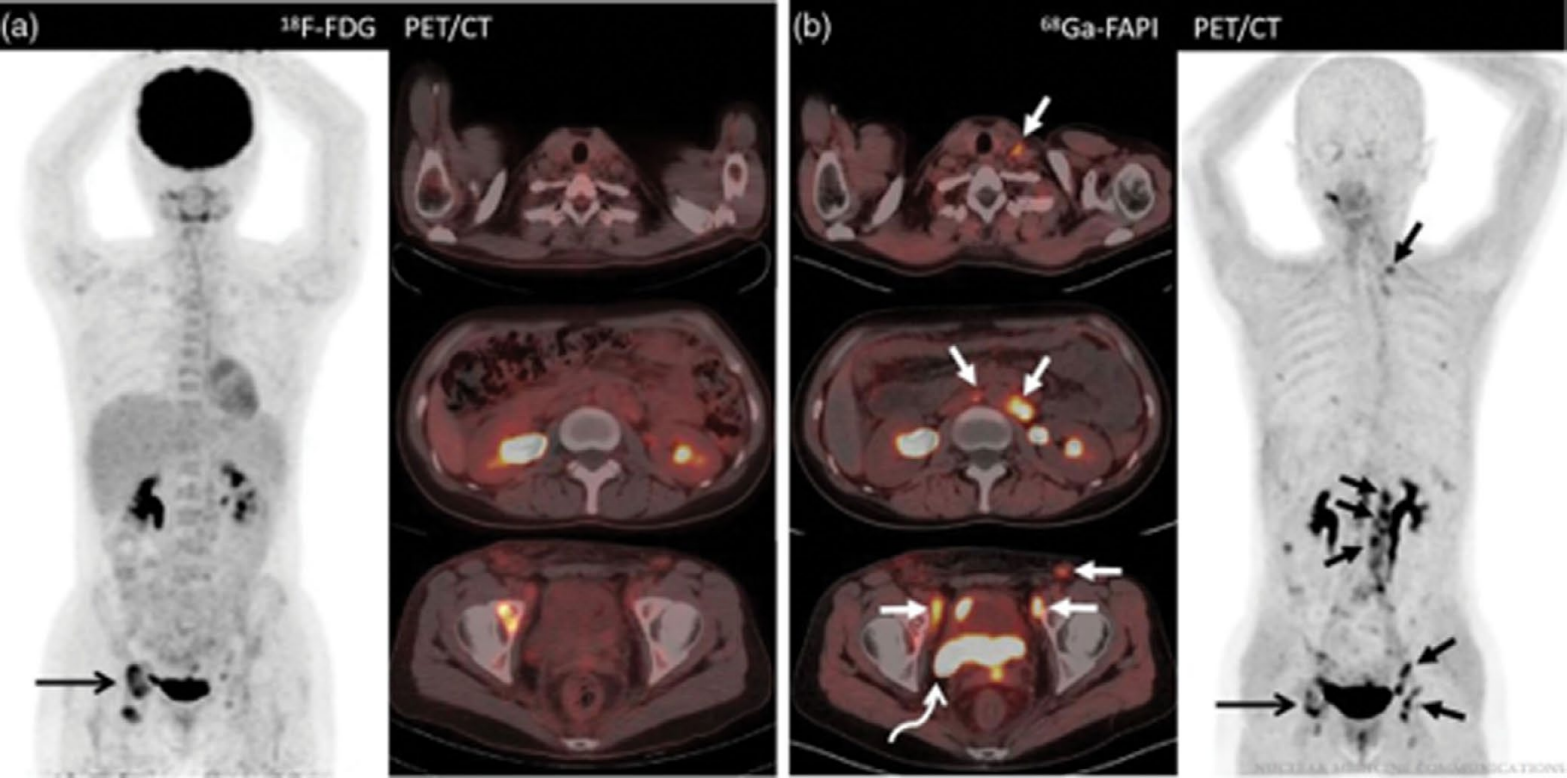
[^18^F]F‐FDG versus [^68^Ga]Ga‐FAPI‐04 positron emission tomography. From Zheng et al. [58] A 35‐year‐old woman who previously underwent surgery for poorly differentiated mucinous ovarian adenocarcinoma. Fluorine‐18‐fluorodeoxyglucose (^18^F‐FDG) positron emission tomography/computed tomography (PET/CT) (a) and gallium‐68‐labelled fibroblast activation protein inhibitor (FAPI)‐04 PET/CT (b) demonstrate intense uptake in the right ilium (long arrow). ^18^F‐FDG PET/CT showed no abnormal uptake throughout the body, whilst ^68^Ga‐FAPI PET/CT showed increased uptake in a cervical lymph node, the retroperitoneal lymph nodes, and the pelvic site of recurrence (bent arrow). Subsequent biopsy of the cervical node found metastatic ovarian serous carcinoma. Copyright 2022 The Author(s). Published by Wolters Kluwer Health Inc.
